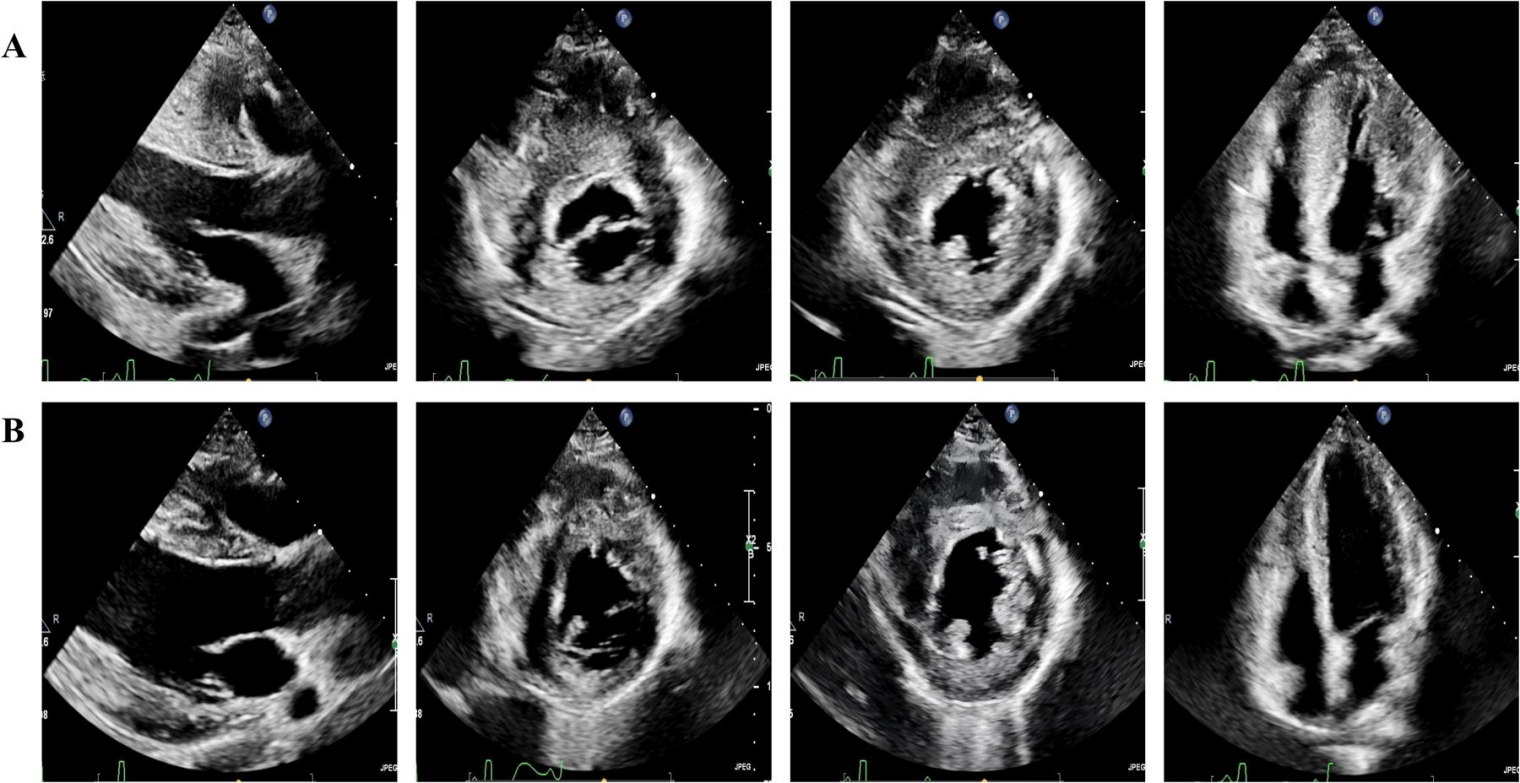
Transthoracic echocardiography. (A) Preoperative transthoracic echocardiography demonstrates severe left ventricular hypertrophy, right ventricular free wall hypertrophy, and a small LVEDV in parasternal long axis of left ventricule, parasternal short axis, and apical four-chamber view. (B) A 6-month postoperative transthoracic echocardiography, compared with (A), reveals significant thinning of both the left ventricular and right ventricular free wall myocardium, alongside a marked increase in LVEDV.
